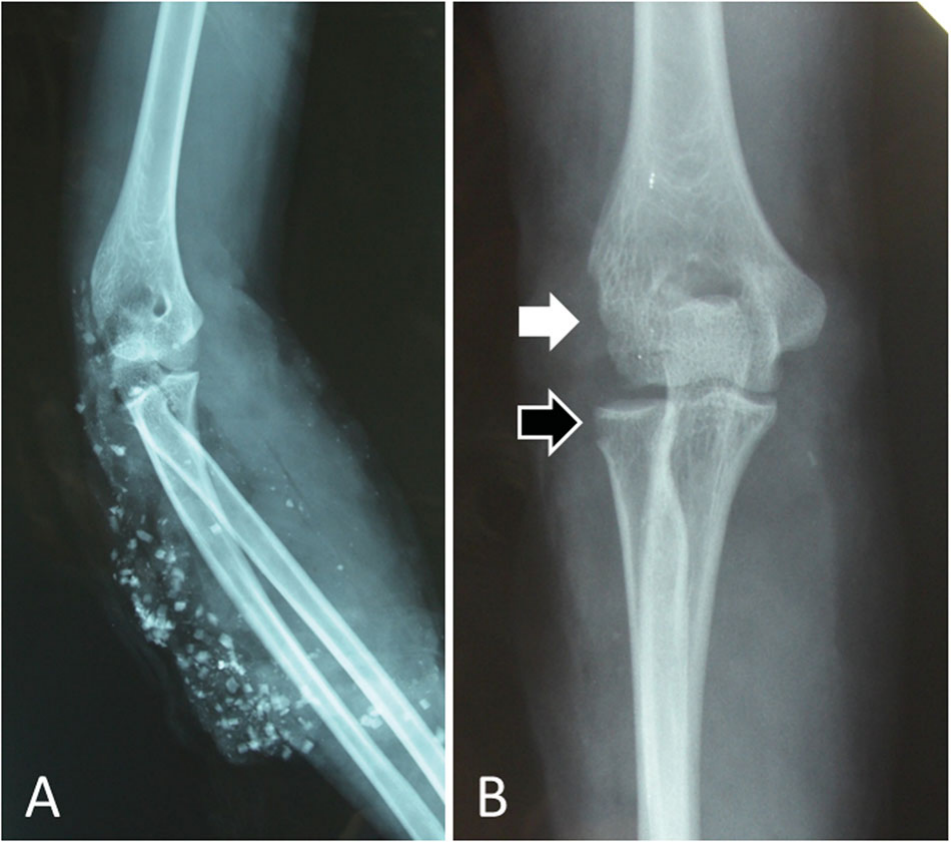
Radiographs of the patient in Case 2. (A) Radiograph taken immediately after the injury. The arm and elbow were contaminated with several windshield pieces. (B) Radiograph taken after debridement. The white arrow indicates deficient humeral condyles, while the black arrow indicates radial head defects.
